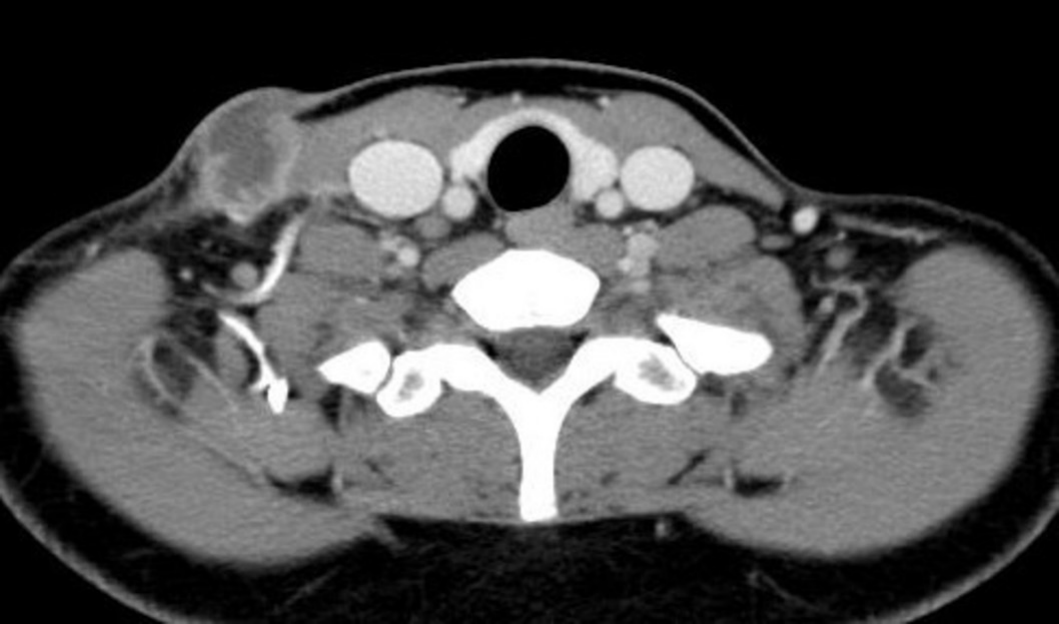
Contrast‐enhanced CT showing an enlarged right cervical lymph node with low internal attenuation.
